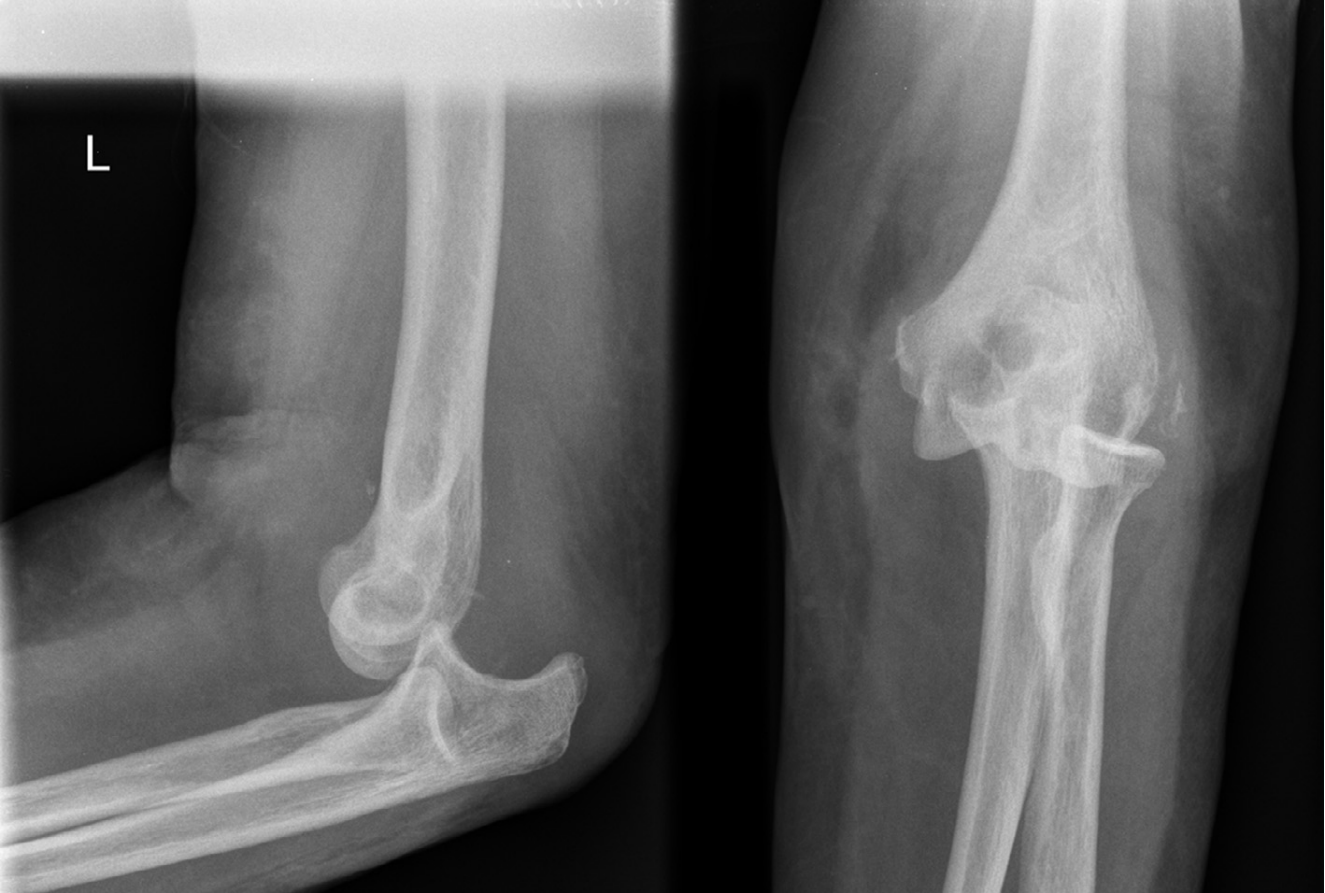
Elbow AP and lateral radiographs demonstrating failure of first reconstruction.
